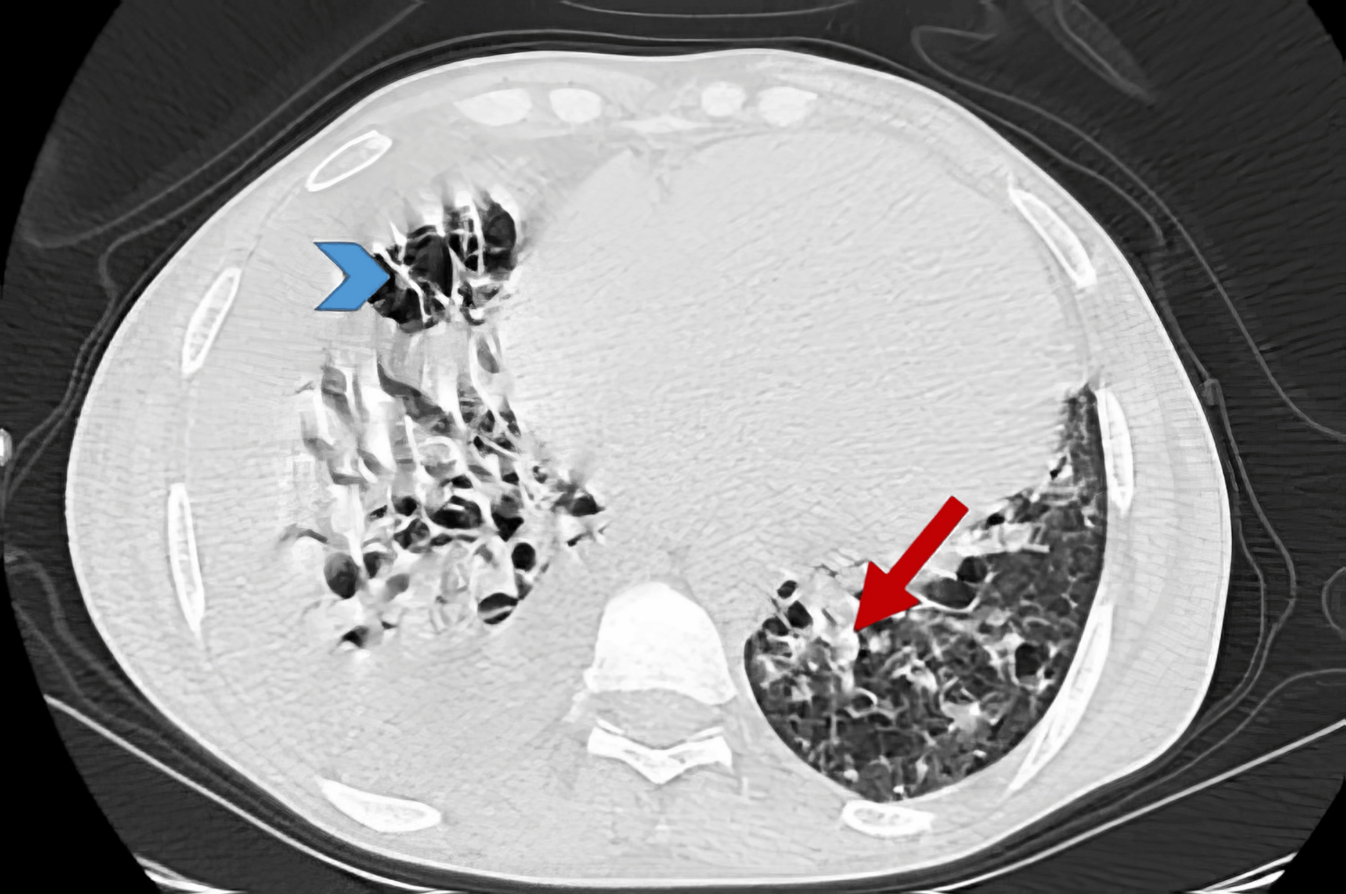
Axial CT scan image in lung window shows bilateral cystic bronchiectasis (blue arrow head) predominantly in the lower lobes with mucoid impactions (red arrow).
